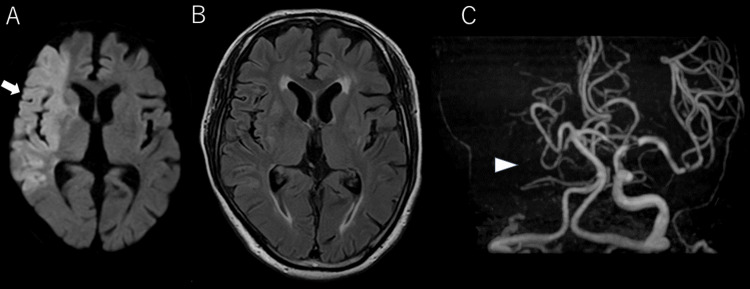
Initial MRI and MRA (A) Initial brain MRI diffusion-weighted images showing brain infarction in the right middle cerebral artery territory (arrow). (B) Fluid-attenuated inversion recovery images showing no ischemic changes. (C) MRA indicating occlusion of the right internal carotid artery (arrowhead). MRI, magnetic resonance imaging; MRA, magnetic resonance angiography
